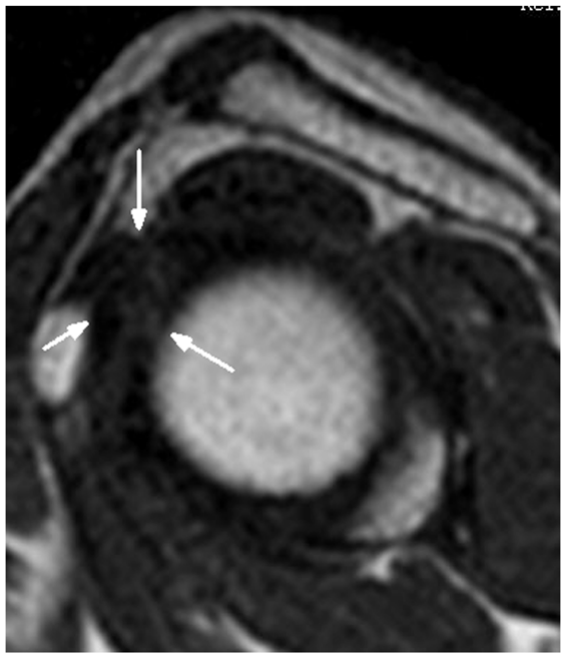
A 54-year-old female normal volunteer. Sagittal oblique, T1-weighted image (TR/TE = 550 ms/15 ms) shows that the distinct fatty tissue surrounding the CHL had disappeared , and the CHL could not be measured (arrows).
